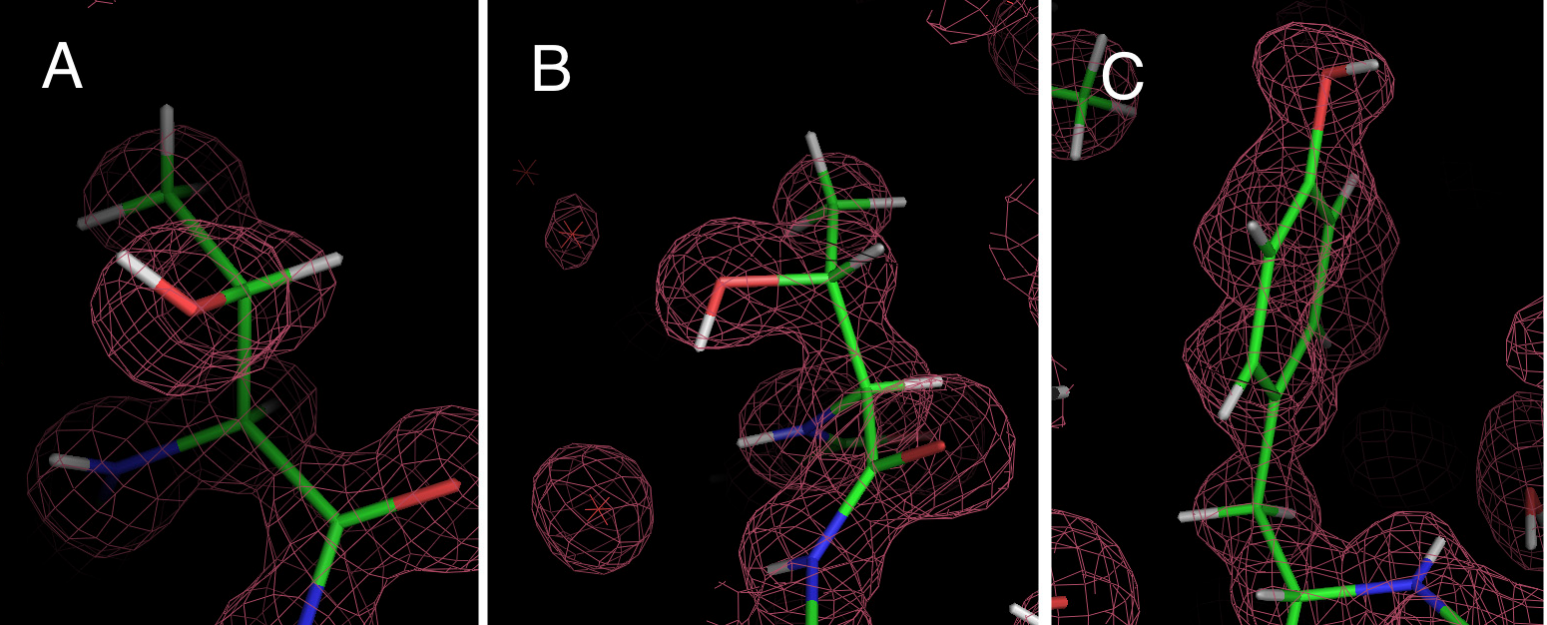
Examples of the poor fits of the hydrogen positions to the 2F_0_-F_c_electron-density map (at 0.5σ contour level) in high-resolution X-ray structures. These conformations are refinement artifacts (A) Thr-A41 [1C75] at χ2 = -120°, (B) Thr-A104 [1JM1] at χ2 = 0°; and (C) Tyr-A3 [1RW1] at χ3 = 60°.
